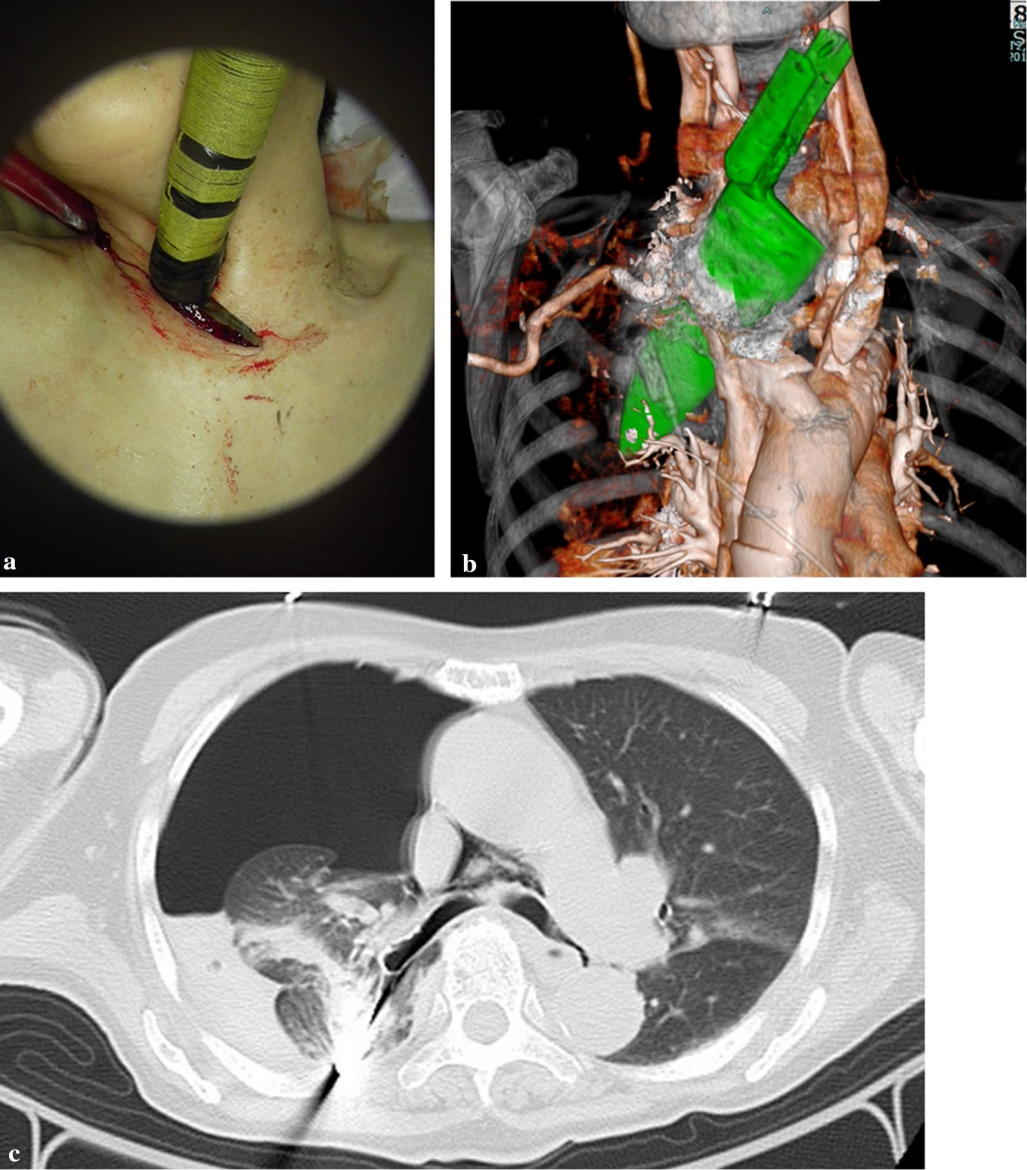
Preoperative image. a A broad-blade kitchen knife penetrating the neck. b Three-dimensional reconstruction from the preoperative computed tomography scan. The knife pierced through the left side of the right brachiocephalic vein and into the right thoracic cavity. c Chest computed tomography showed a right hemopneumothorax with collapse of about half of the right lung and a small amount of thoracic effusion
